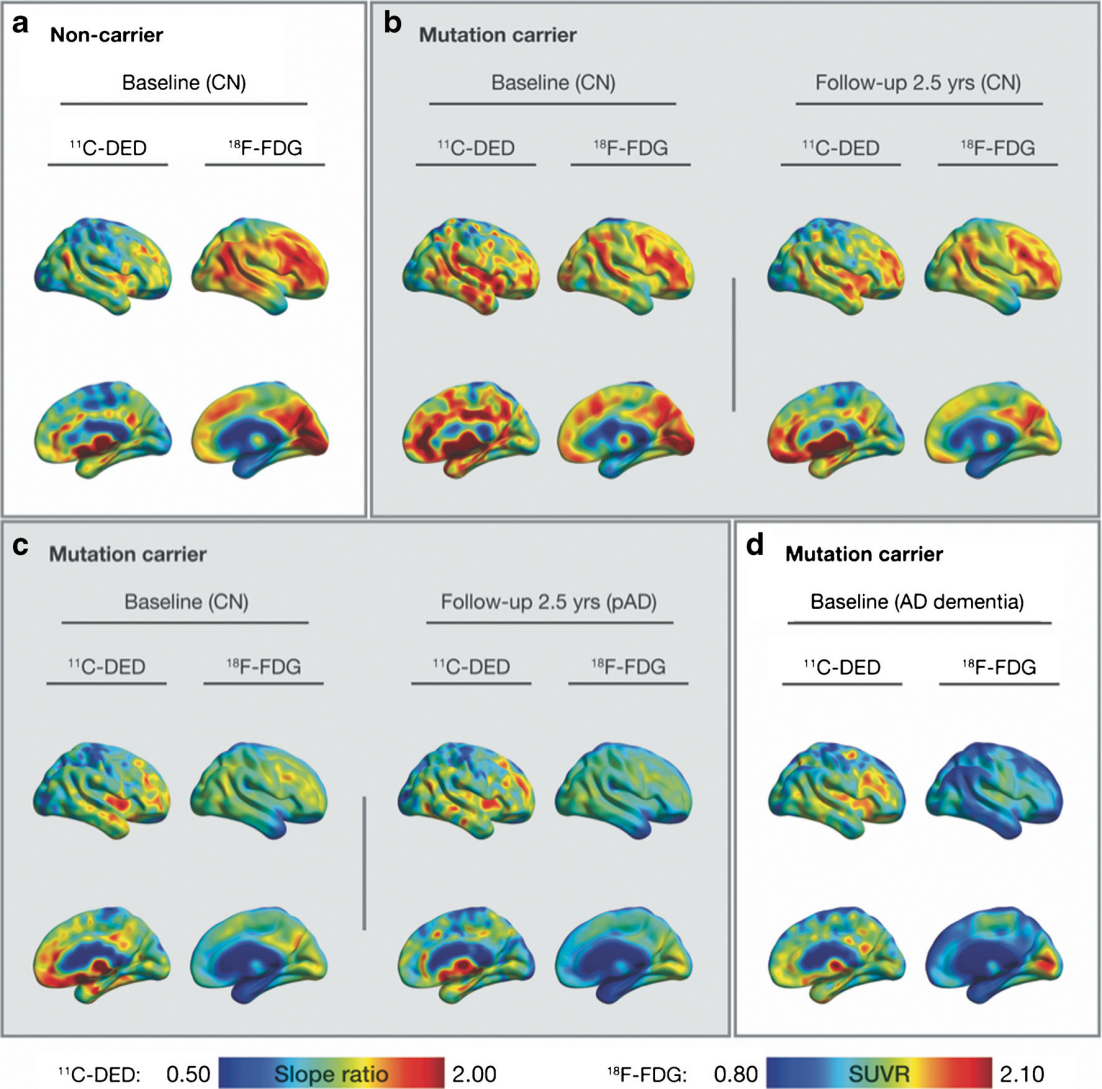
Representative ^11^C-DED and ^18^F-FDG PET images. a A noncarrier. b A mutation carrier who was asymptomatic both at baseline (around two decades before symptom onset) and at the 2.5-year follow-up. c A mutation carrier who was asymptomatic at baseline and who had converted to prodromal AD at the 2.5-year follow-up. d A mutation carrier who was symptomatic with a diagnosis of AD dementia about 6 years after onset. *AD* Alzheimer’s disease, *CN* cognitively normal, ^*11*^*C-DED*
